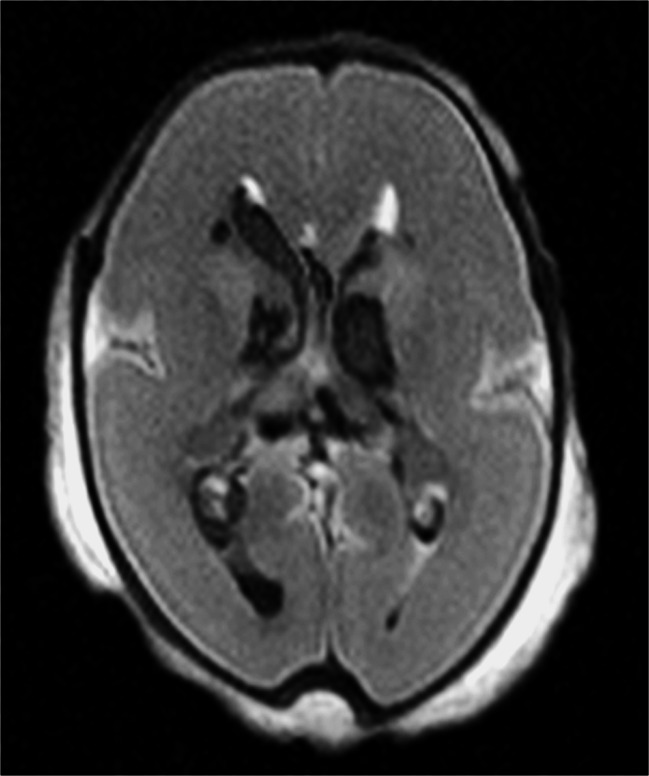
Postmortem MRI of the foetal brain. Axial T_2_-weighted postmortem MR image of the brain in a 23-week foetus shows bilateral intraventricular and periventricular haemorrhage
